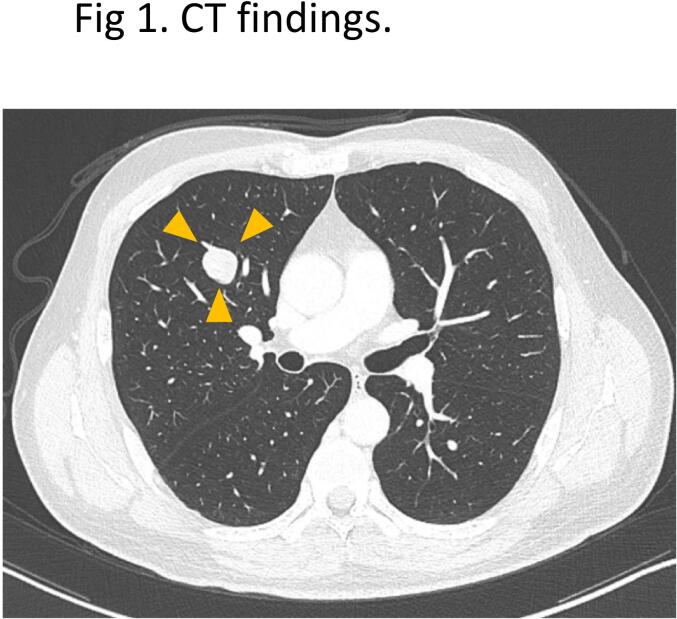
CT findings. CT of the chest shows a well-defined bordering mass of 2.5 × 2.0 cm in S3 of the right lung. The mass extent is indicated by yellow arrow heads. (For interpretation of the references to colour in this figure legend, the reader is referred to the web version of this article.)
